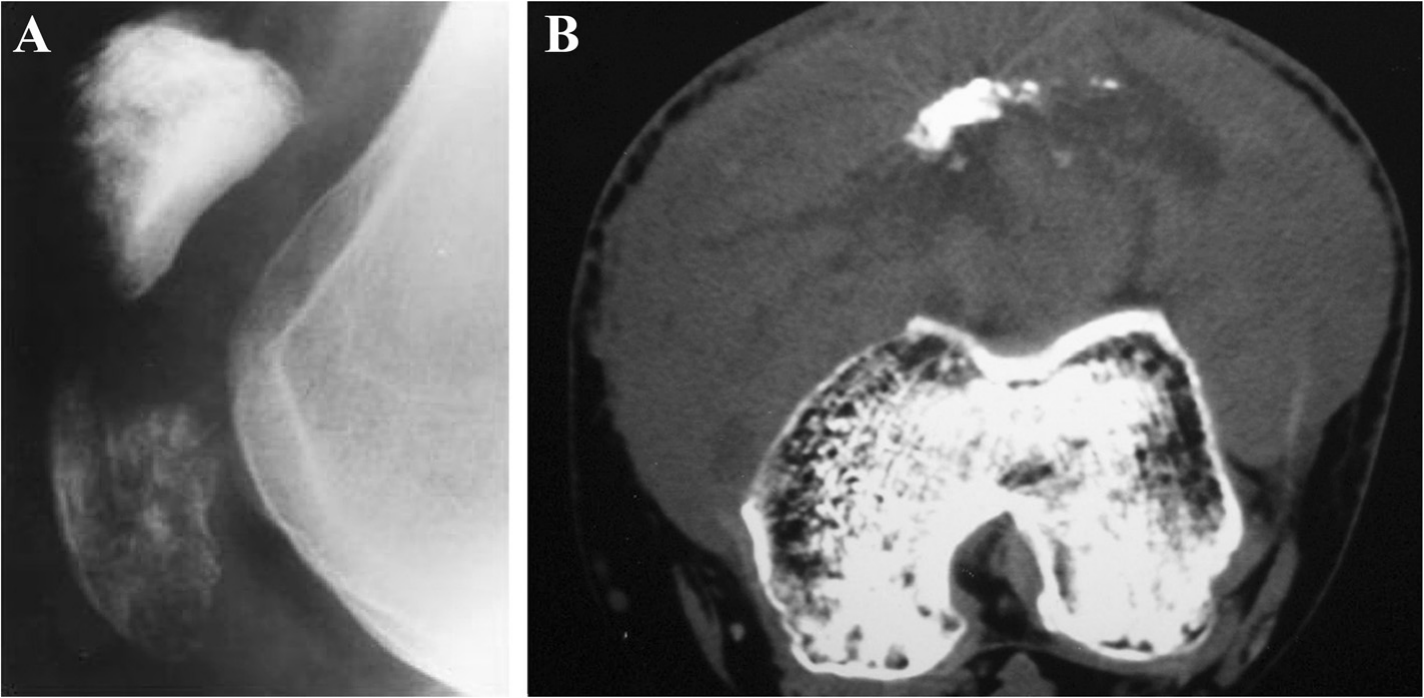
Patellar lymphoma with a pathologic fracture. (A) On radiographs, the patella consists of a sclerotic proximal portion and a lytic distal portion. (B) Permeative bone destruction, hazy margins, destroyed cortex, large soft tissue mass surrounding the patella, and joint involvement are shown on CT images.
